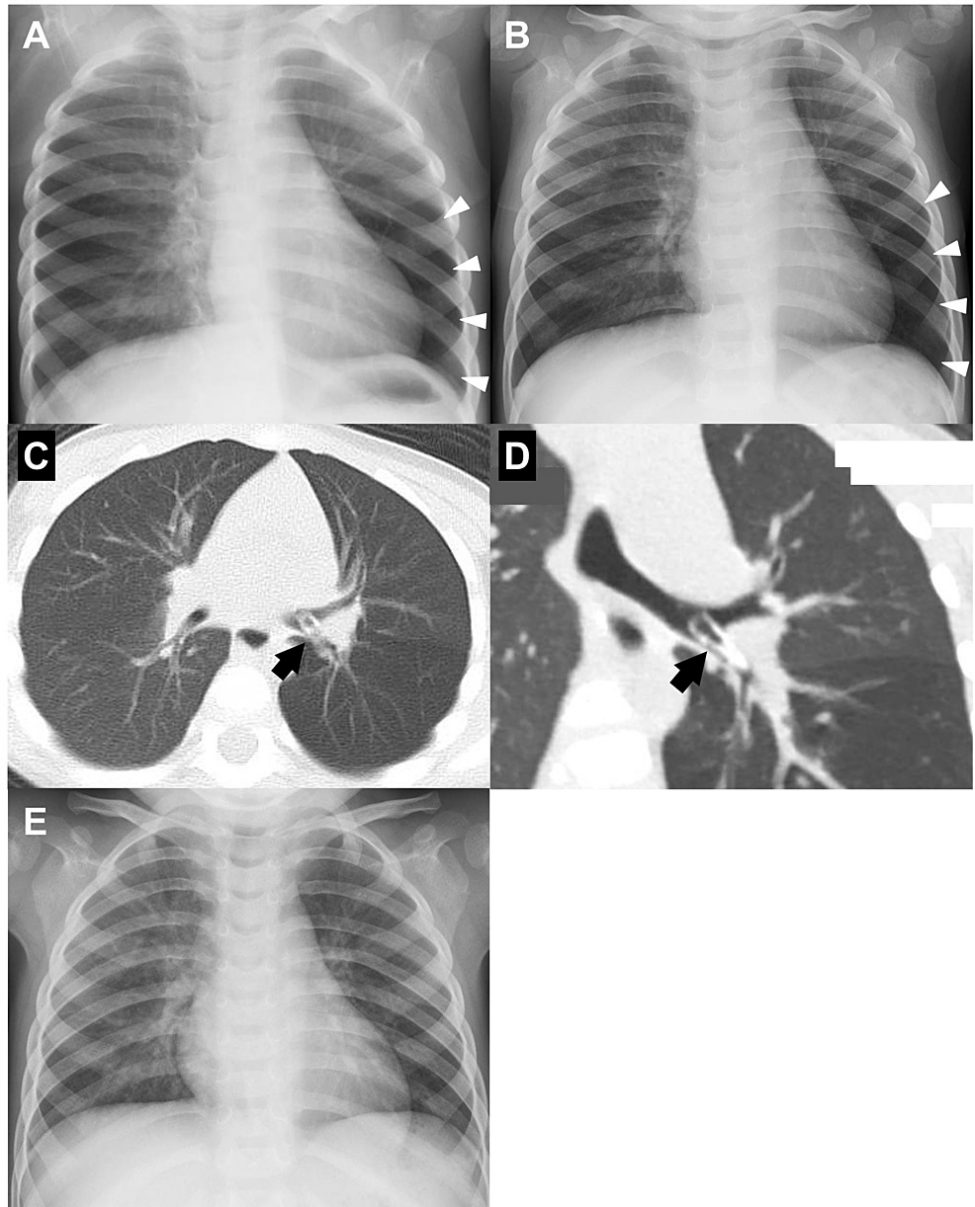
CXR showing slight emphysema of the left lower lung. (A) CXR on the first day of visiting the hospital. (B) CXR four days later. (C) Axial CT showing a plastic foreign body at the left inferior lobar bronchus (black arrow). (D) Coronal CT showing the cranial foreign body (black arrow). (E) CXR after removing the foreign body, in which partial emphysema disappeared. CXR, chest X-ray; CT, computed tomography
